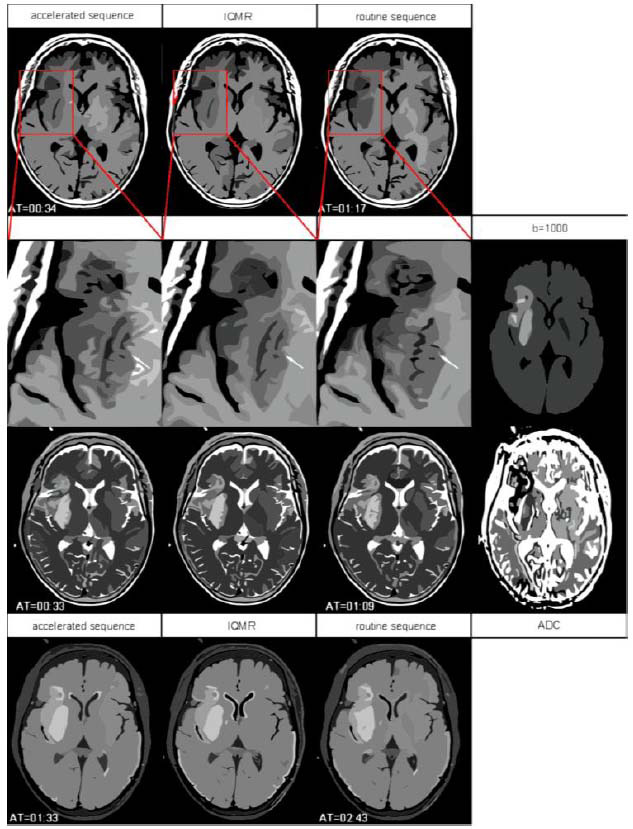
Acute ischemic stroke in a 67-year-old female patient. Compared to routine sequences, accelerated sequences shortened examination times by 55.84% (T1WI), 52.17% (T2WI), and 42.95% (T2-FLAIR). However, accelerated sequences showed increased image noise and lower overall quality. After IQMR post-processing, lesion conspicuity, internal lesion detail, and overall image quality significantly improved. IQMR images provided better internal lesion display (white arrow) and equivalent lesion conspicuity and overall image quality compared to routine sequences.
